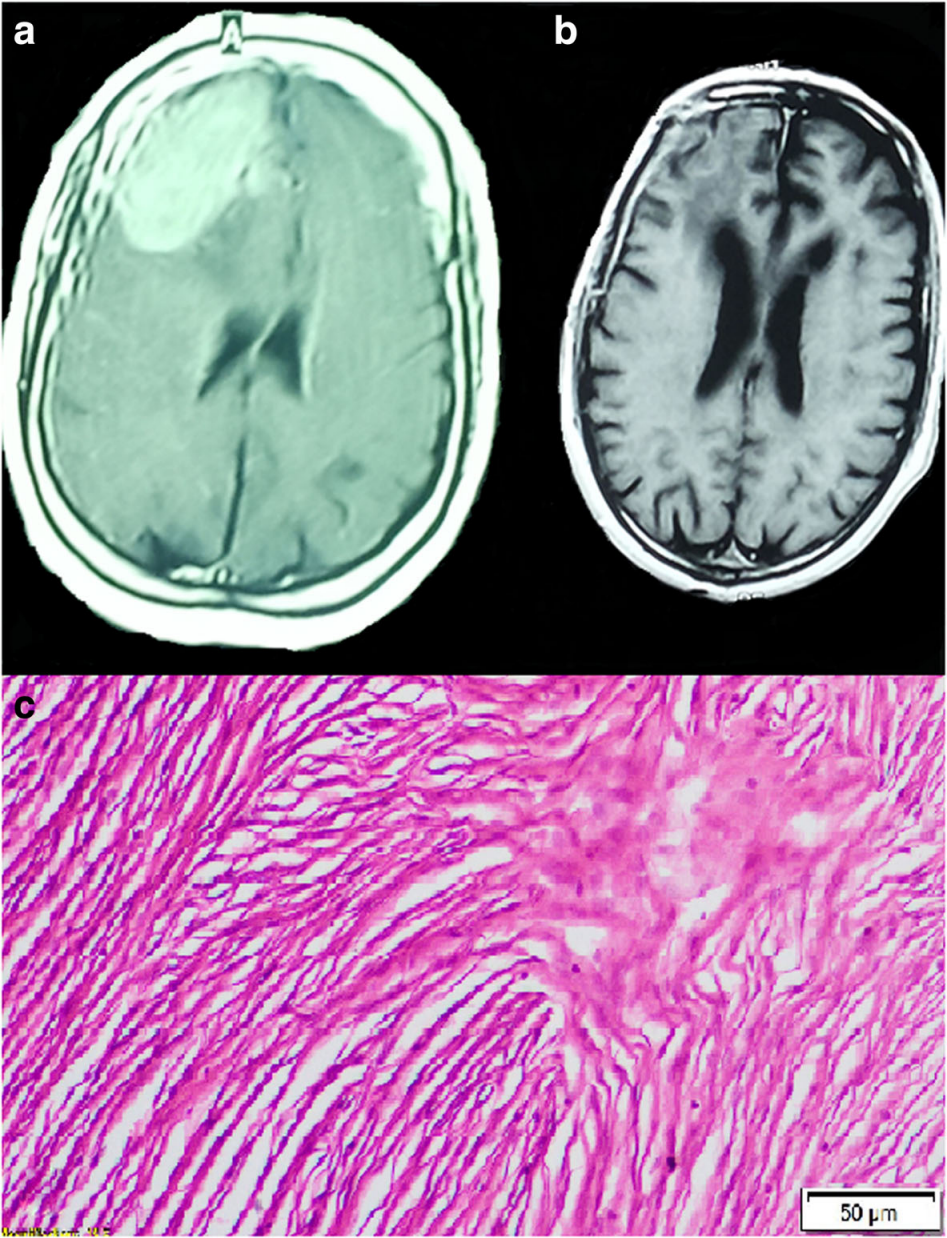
Convexity meningioma. a Preoperative image, MRI with contrast (axial) showed mass lesion in the right frontal region. The tumor was surrounded by mild edema. b One year postoperative MRI with contrast (axial) revealed complete removal of the mass. Geriatric Scoring System (GSS) score = 16. c The slide represented fibrotic meningioma, WHO grade I
